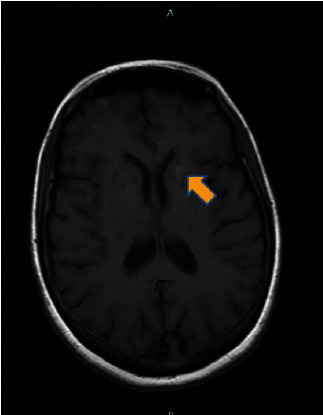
The axial T1-weighted image shows an enhancing nodule of the left caudate.
